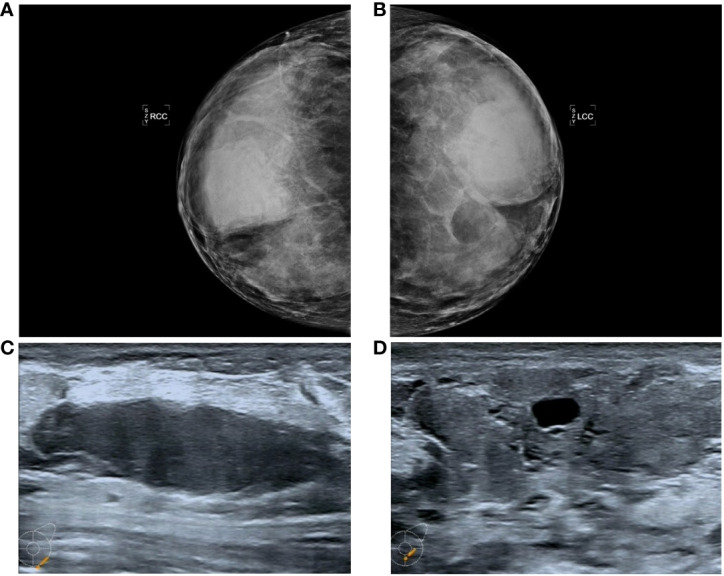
Manifestations (A, B) in the PASH image showed changes in the double milk molybdenum target, which showed an oval isondense mass. (C, D) show ultrasound changes in both breasts, manifested as hypoechoic nodules with clear boundaries and cystic changes.
